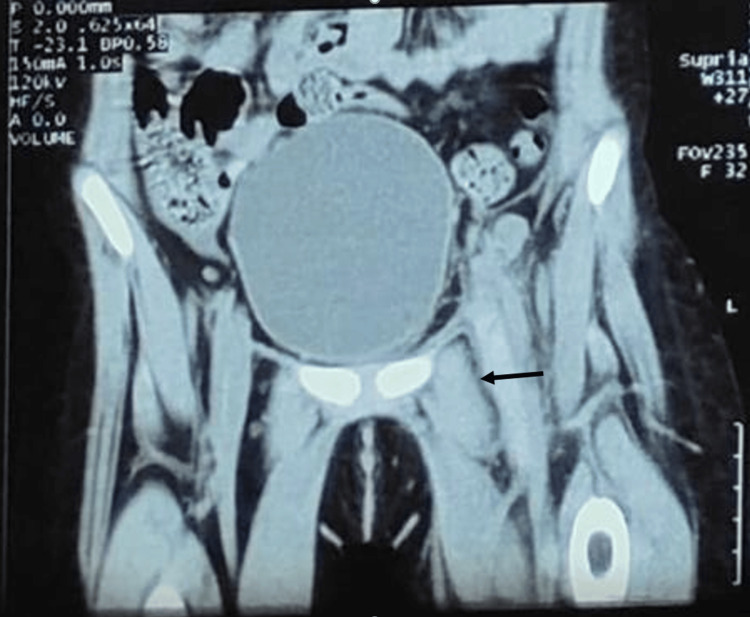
Coronal section CT scan Distended and hyperdense common external iliac, and femoral veins, suggesting thrombosis.
